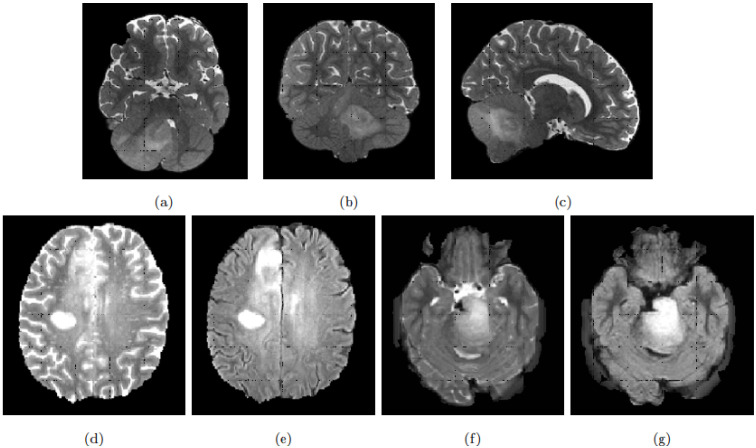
Top row: Example of T2w MRI scan of a patient with DIPG tumour extending beyond the pons. (a) Axial, (b) Coronal and (c) Sagittal slices. Bottom row: Example of glioblastoma and DIPG MRI scans, in axial slices. (d) glioblastoma T2w, (e) glioblastoma FLAIR, (f) DIPG T2w and (g) DIPG FLAIR.
